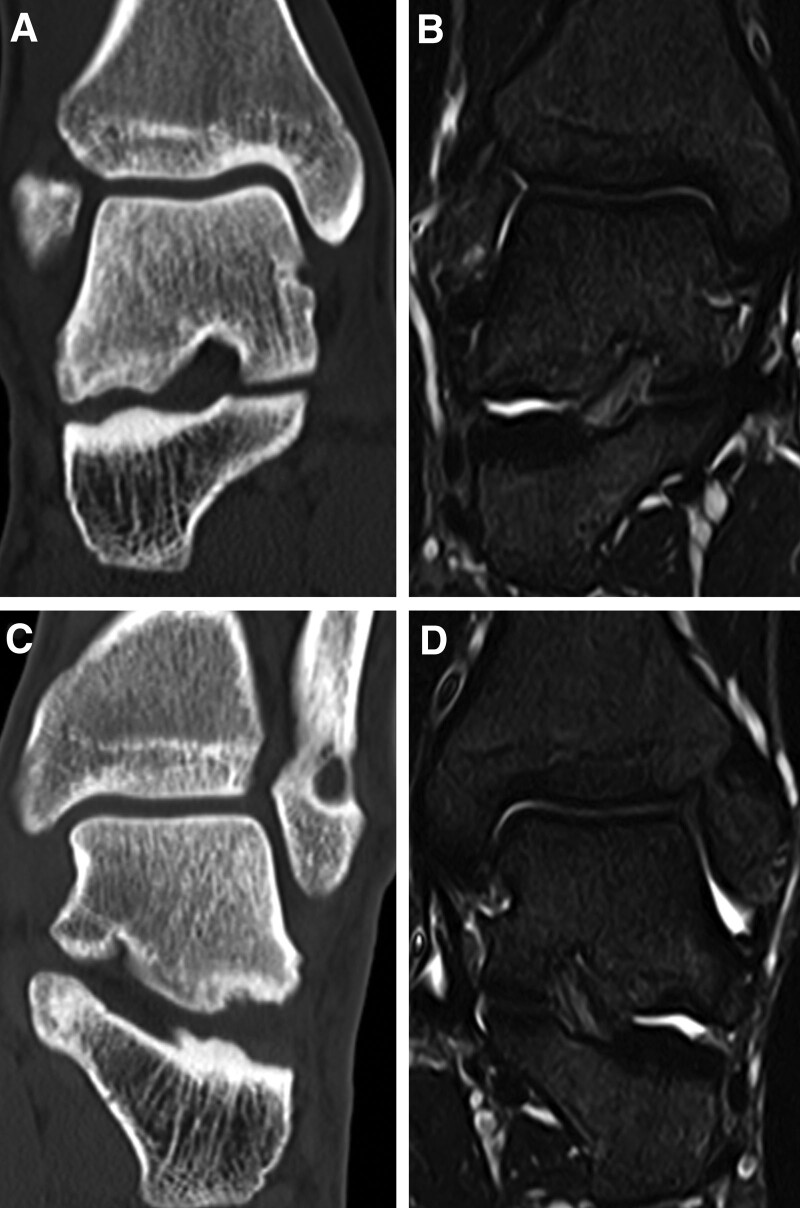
Postoperative computed tomography of the right (A) and the left (C) feet reveal remodeling of subchondral bones; magnetic resonance imaging of the right (B) and the left (D) feet reveal disappearances of bone mallow edemas on lateral process of the talus (arrows).
